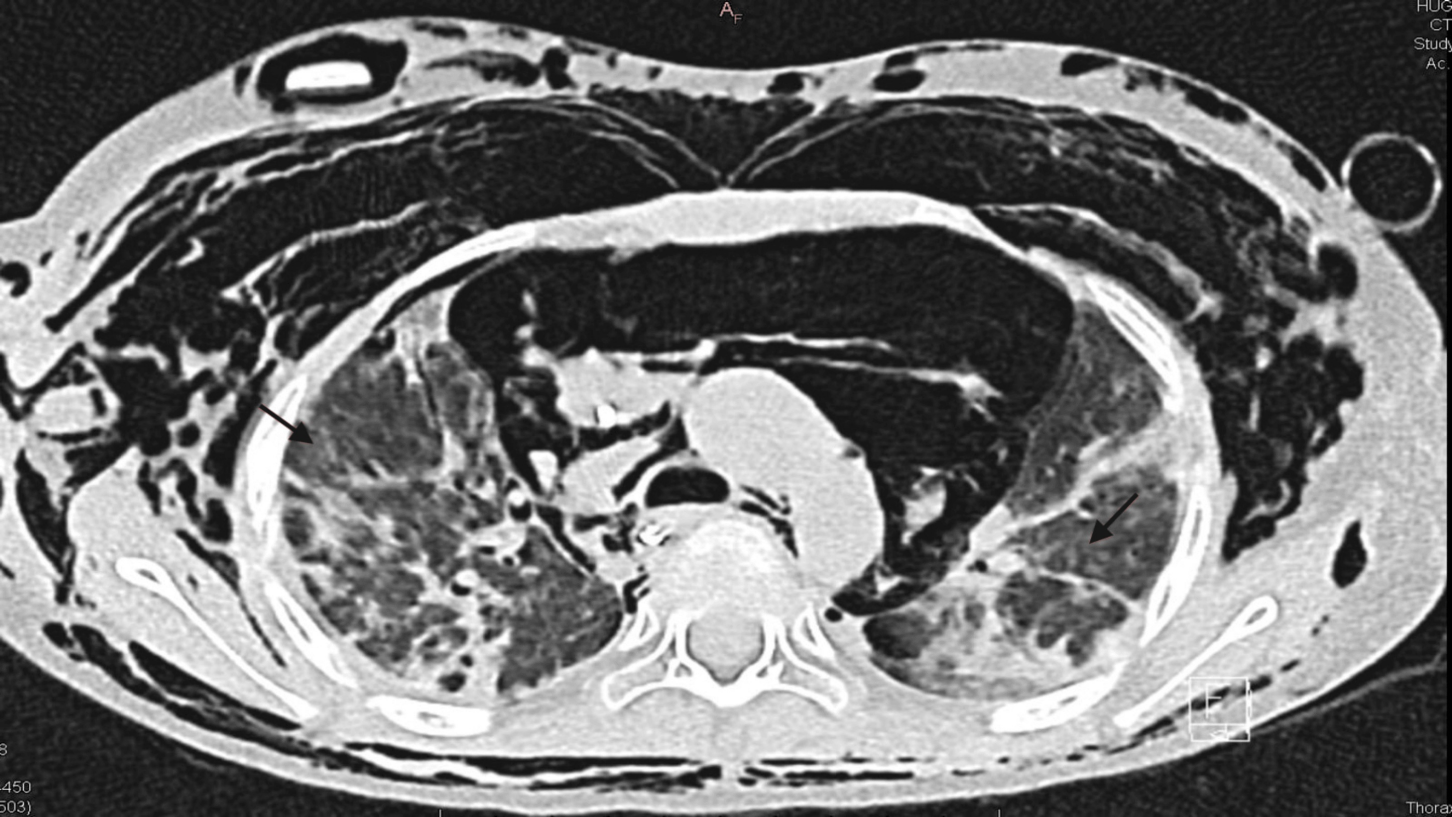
Chest CT scan showing fibrotic changes in the lungs
